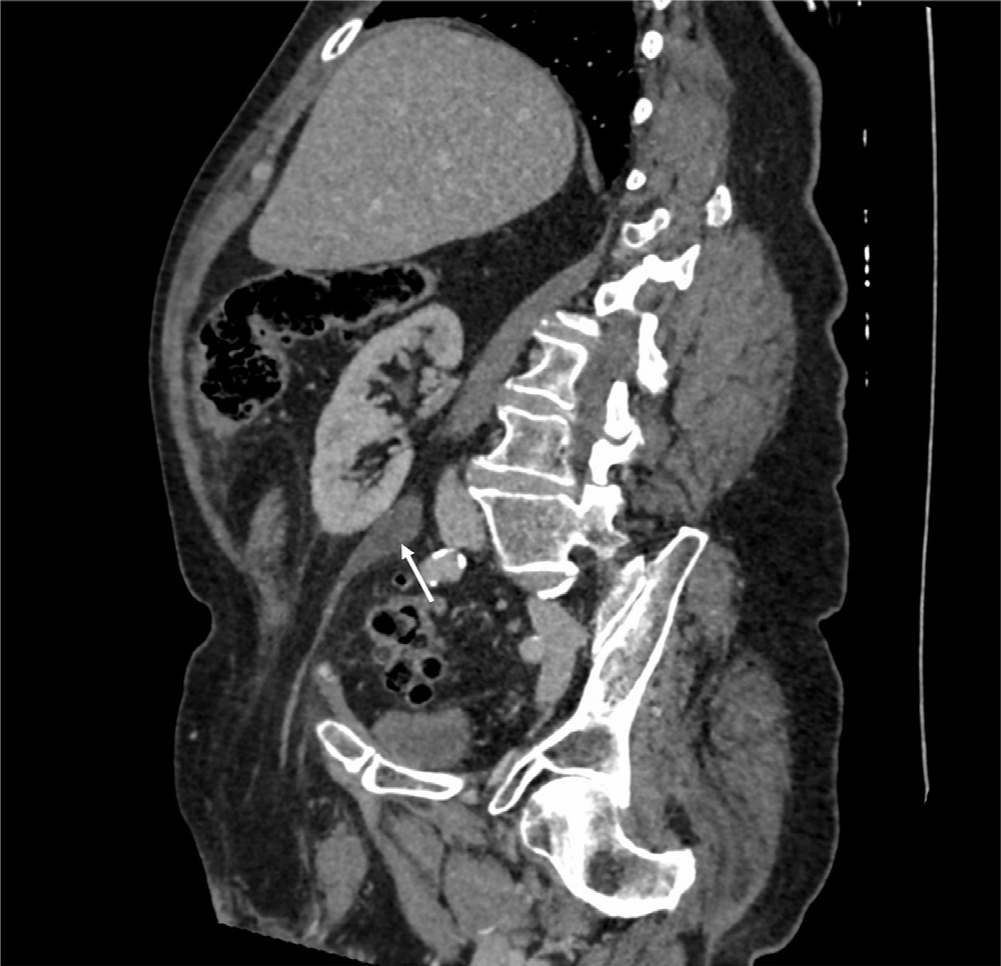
The patient's prior CT, showing milder hydronephrosis/hydroureter (white arrow) and an otherwise normal kidney.
